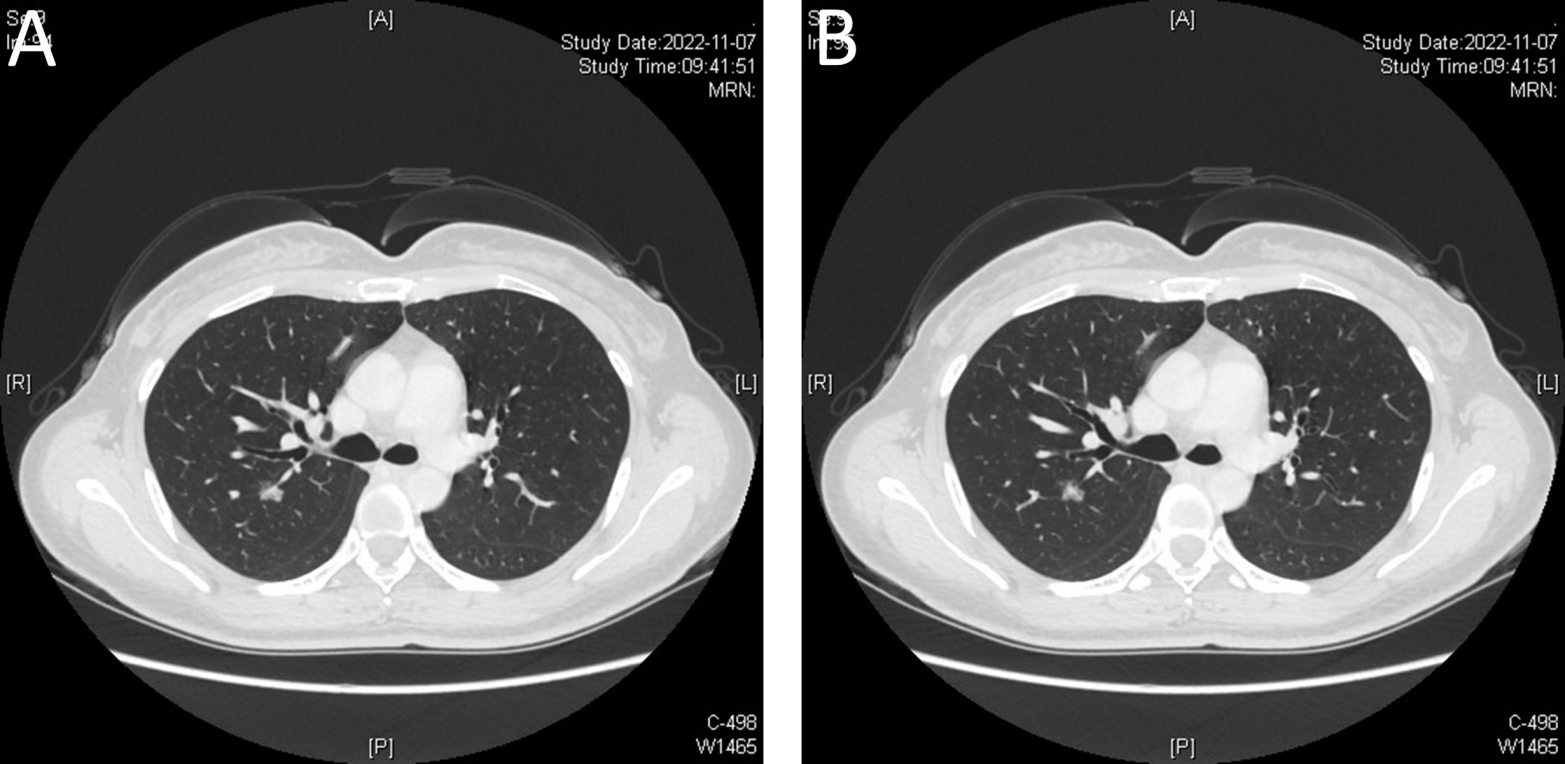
(A, B) Enhanced computed tomography (CT) indicates a mixed ground glass opacity. Blood vessels can be seen passing through the lesion, and the enhanced scan shows that the solid components of the lesion are few, and the degree of enhancement is unclear.
